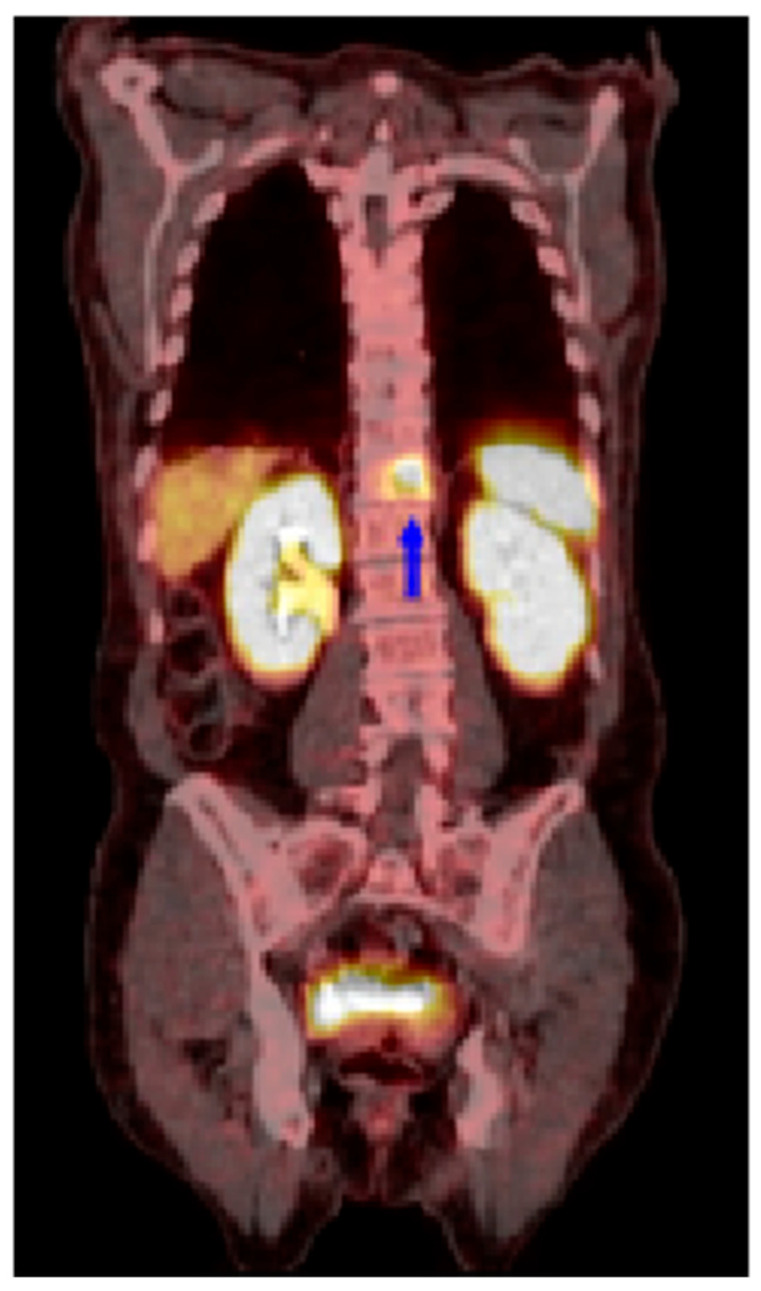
A ^68^Ga-DOTATATE PET/CT scan showing SSTR2 positivity in spinal metastases from a patient with HCC. The blue arrow indicates the location of the spinal metastases originating from the liver.
